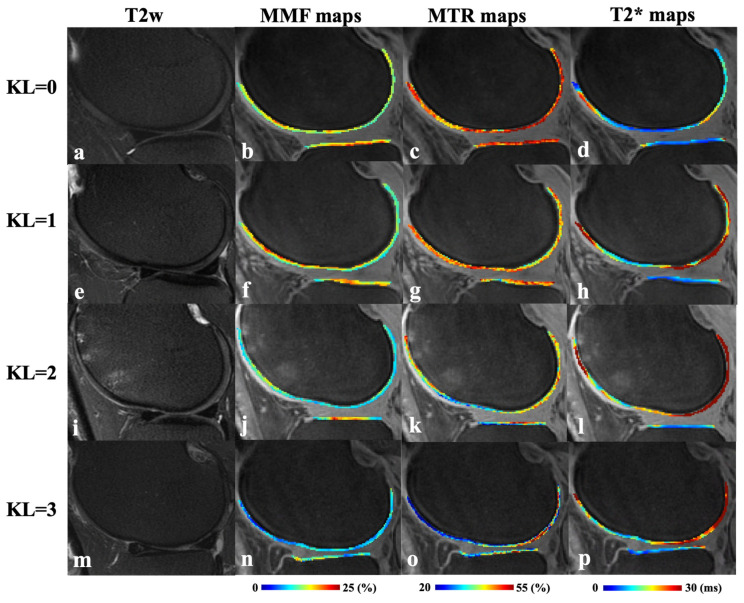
Representative fat-suppressed T2w-FSE (T2w) images (a,e,i,m) and corresponding MMF (b,f,j,n), MTR (c,g,k,o), and T2* (d,h,l,p) maps from four participants with varying degrees of KL scores. Top row (a–d): a 23-year-old male with normal cartilage (KL = 0); second row (e–h): a 57-year-old male with subtle cartilage changes (KL = 1); third row (i–l): a 52-year-old female with mild cartilage degeneration (KL = 2); bottom row (m–p): a 70-year-old female with moderate cartilage degeneration (KL = 3). T2w, T2-weighted fast spin echo; MMF, macromolecular fraction; MTR, magnetization transfer ratio.
